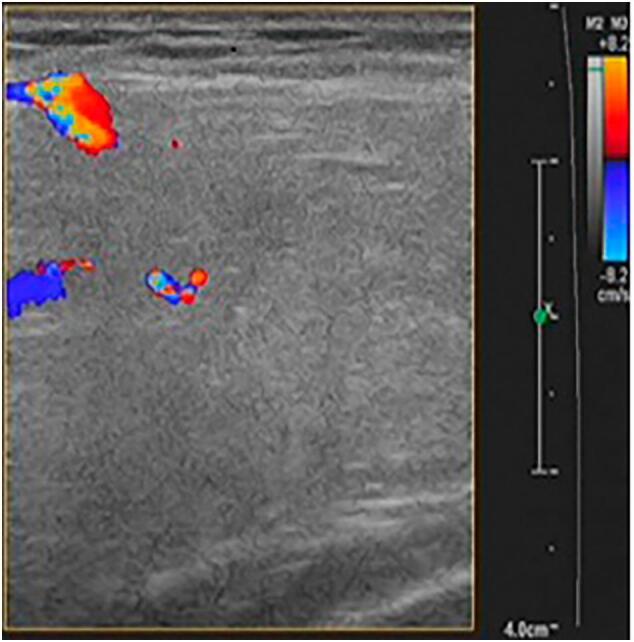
Ultrasonography revealed a hyperechoic area, with a maximum diameter of6 mm, on the upperouter quadrant of the right breast.
